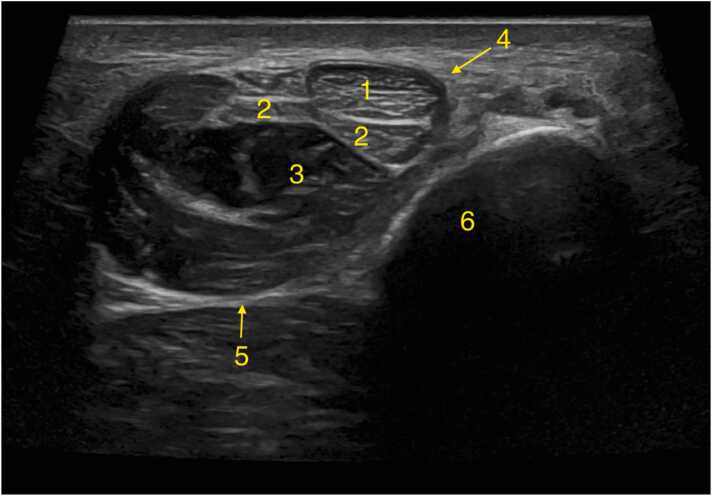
Ultrasound transverse section of the peroneal tendons at the level of the lateral malleolus, slightly superior to the superior peroneal tunnel. The image reveals normal variation of the peroneus brevis tendon with central flattening, accompanied by slight thickening in the medial and lateral regions. 1- Peroneus longus, 2- Peroneus brevis (tendon), 3- Peroneus brevis (muscle), 4- Superior peroneal retinaculum, 5- Crural fascia, 6- Fibula.
